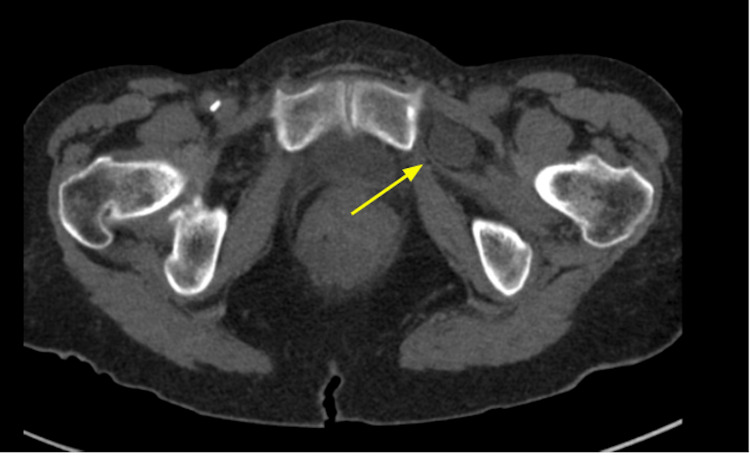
Axial CT demonstrating a left-sided obturator hernia (yellow arrow)
